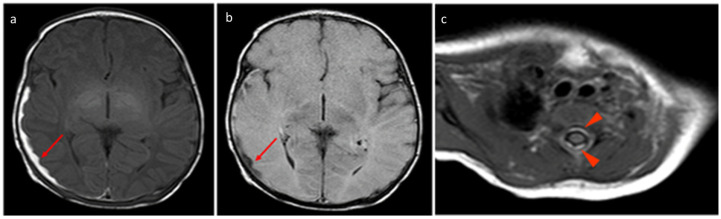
In the same patient in Figure 3, birth-related SDH in an 11-day-old infant born spontaneously:a Axial SE T1-weighted sequence at the level of the basal ganglia—subdural hematoma with occipital and temporal distribution (arrow). (b) FLAIR T2-weighted sequence on the axial plane at the same level as in Figure 4a.The hematoma appears hypointense (arrow) and, considering the hyperintensity on T1, it can be dated as early subacute (intracellular methemoglobin). (c) SE T1-weighted sequence on the axial plane passing through the distal cervical tract confirms (arrowhead) what was described in Figure 4a.
